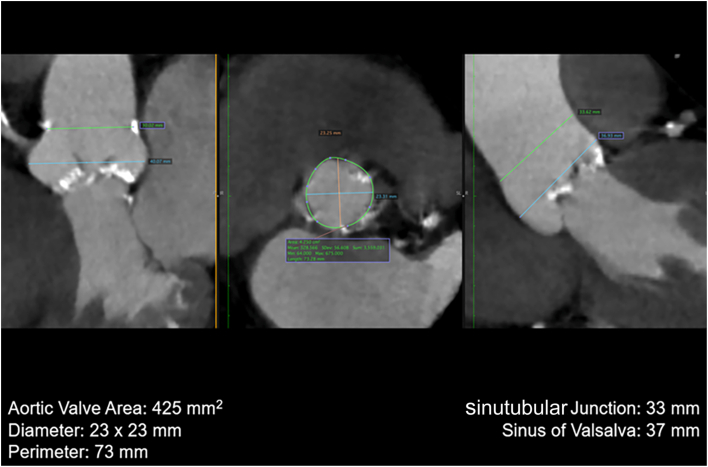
Unplanned, valve-in-valve transcatheter aortic valve replacement in an 85-year-old woman with dyspnea, New York Heart Association functional class III. Transthoracic echocardiography showed a mean aortic gradient of 47 mm Hg and a peak velocity of 4.4 m/s. Computed tomographic angiography detected aortic annular calcification (see image sections above).
